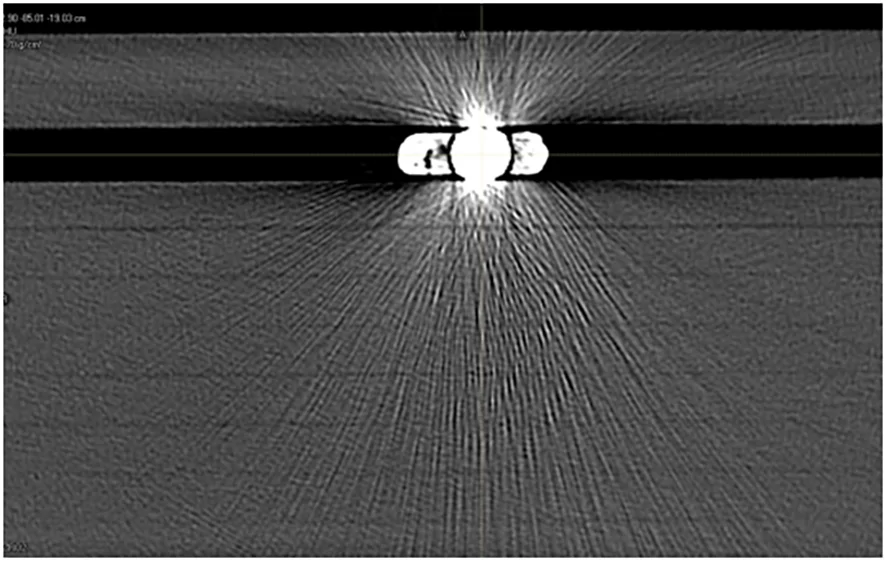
Artifact CT image (ZrO_2_).
